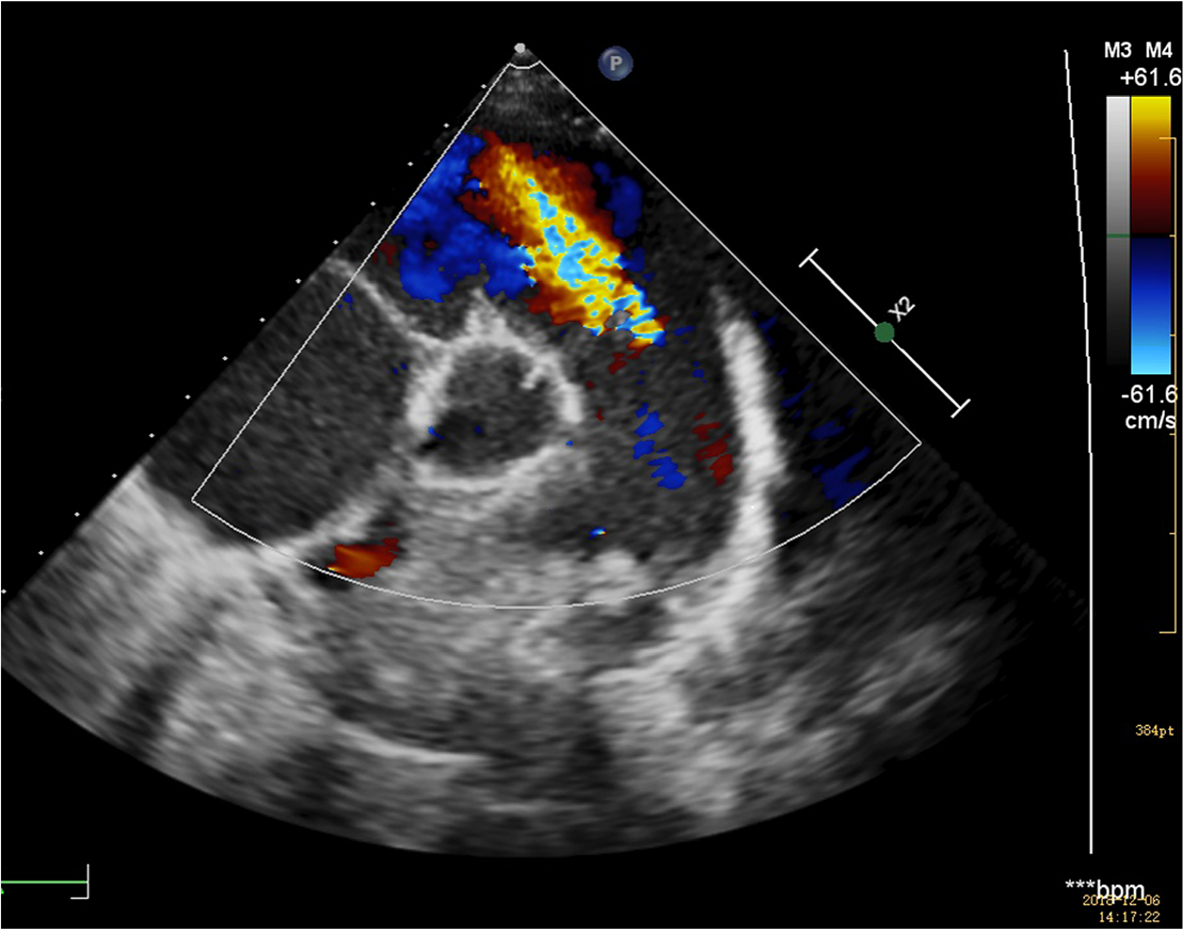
Transthoracic echocardiogram demonstrates widened pulmonary artery trunk and pulmonary regurgitation
